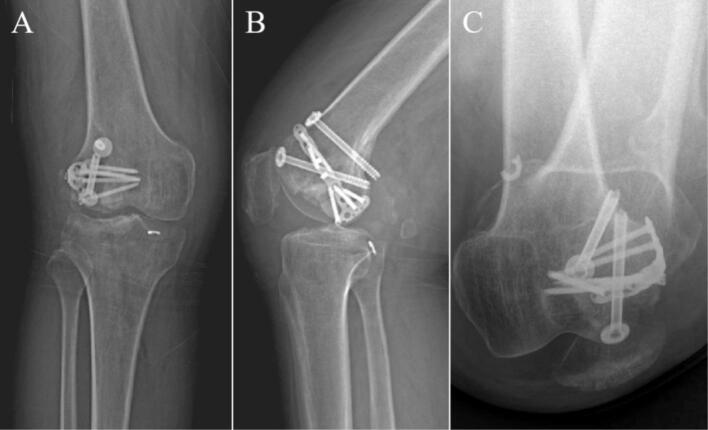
The X-ray images of a second incision for internal fixation at a local hospital. (A) AP view of the right knee. (B) Lateral view of the right knee. (C) Axial view of the right knee.
